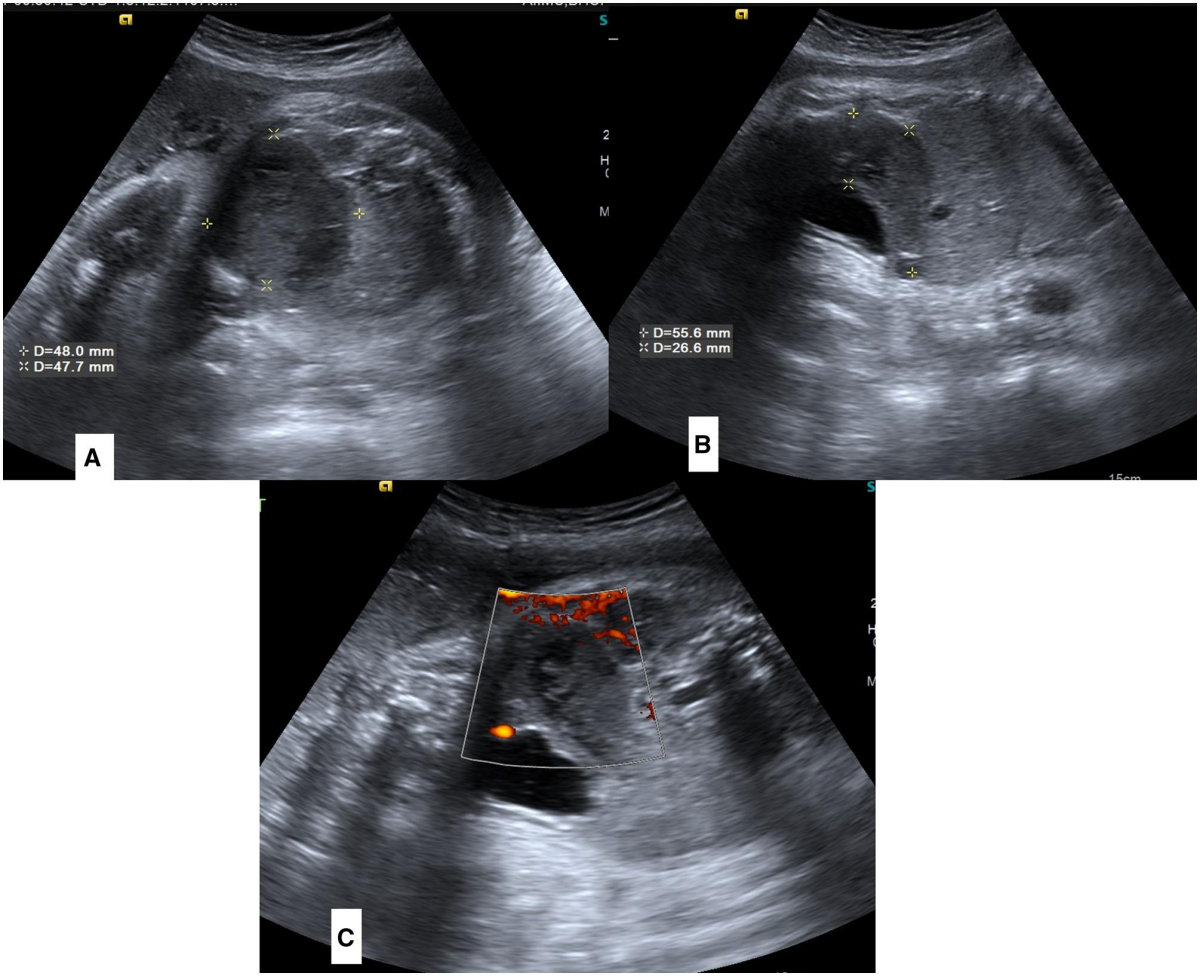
(A–C) Antenatal sonography images of foetal lower abdomen and pelvis. Sagittal (A) and Axial (B) images show a dilated pouch-like structure posterosuperior to urinary bladder (marked by asterisks). Colour doppler images (C) is showing absence of any colour flow in lesion.
